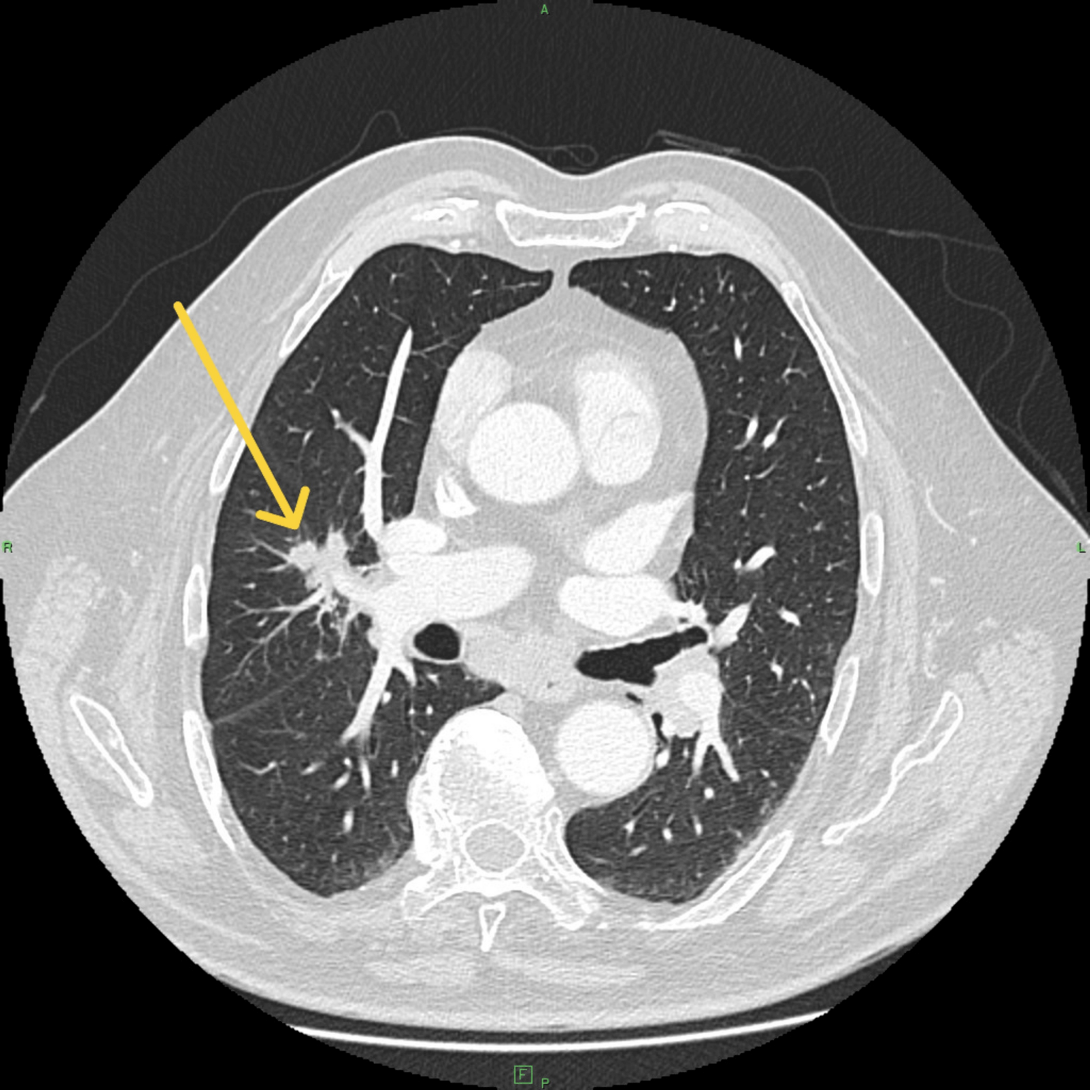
Axial contrast-enhanced CT image demonstrating a 25 × 20 mm irregular parahilar mass in the right upper lobe with spiculated margins, initially suspicious for malignancy.
